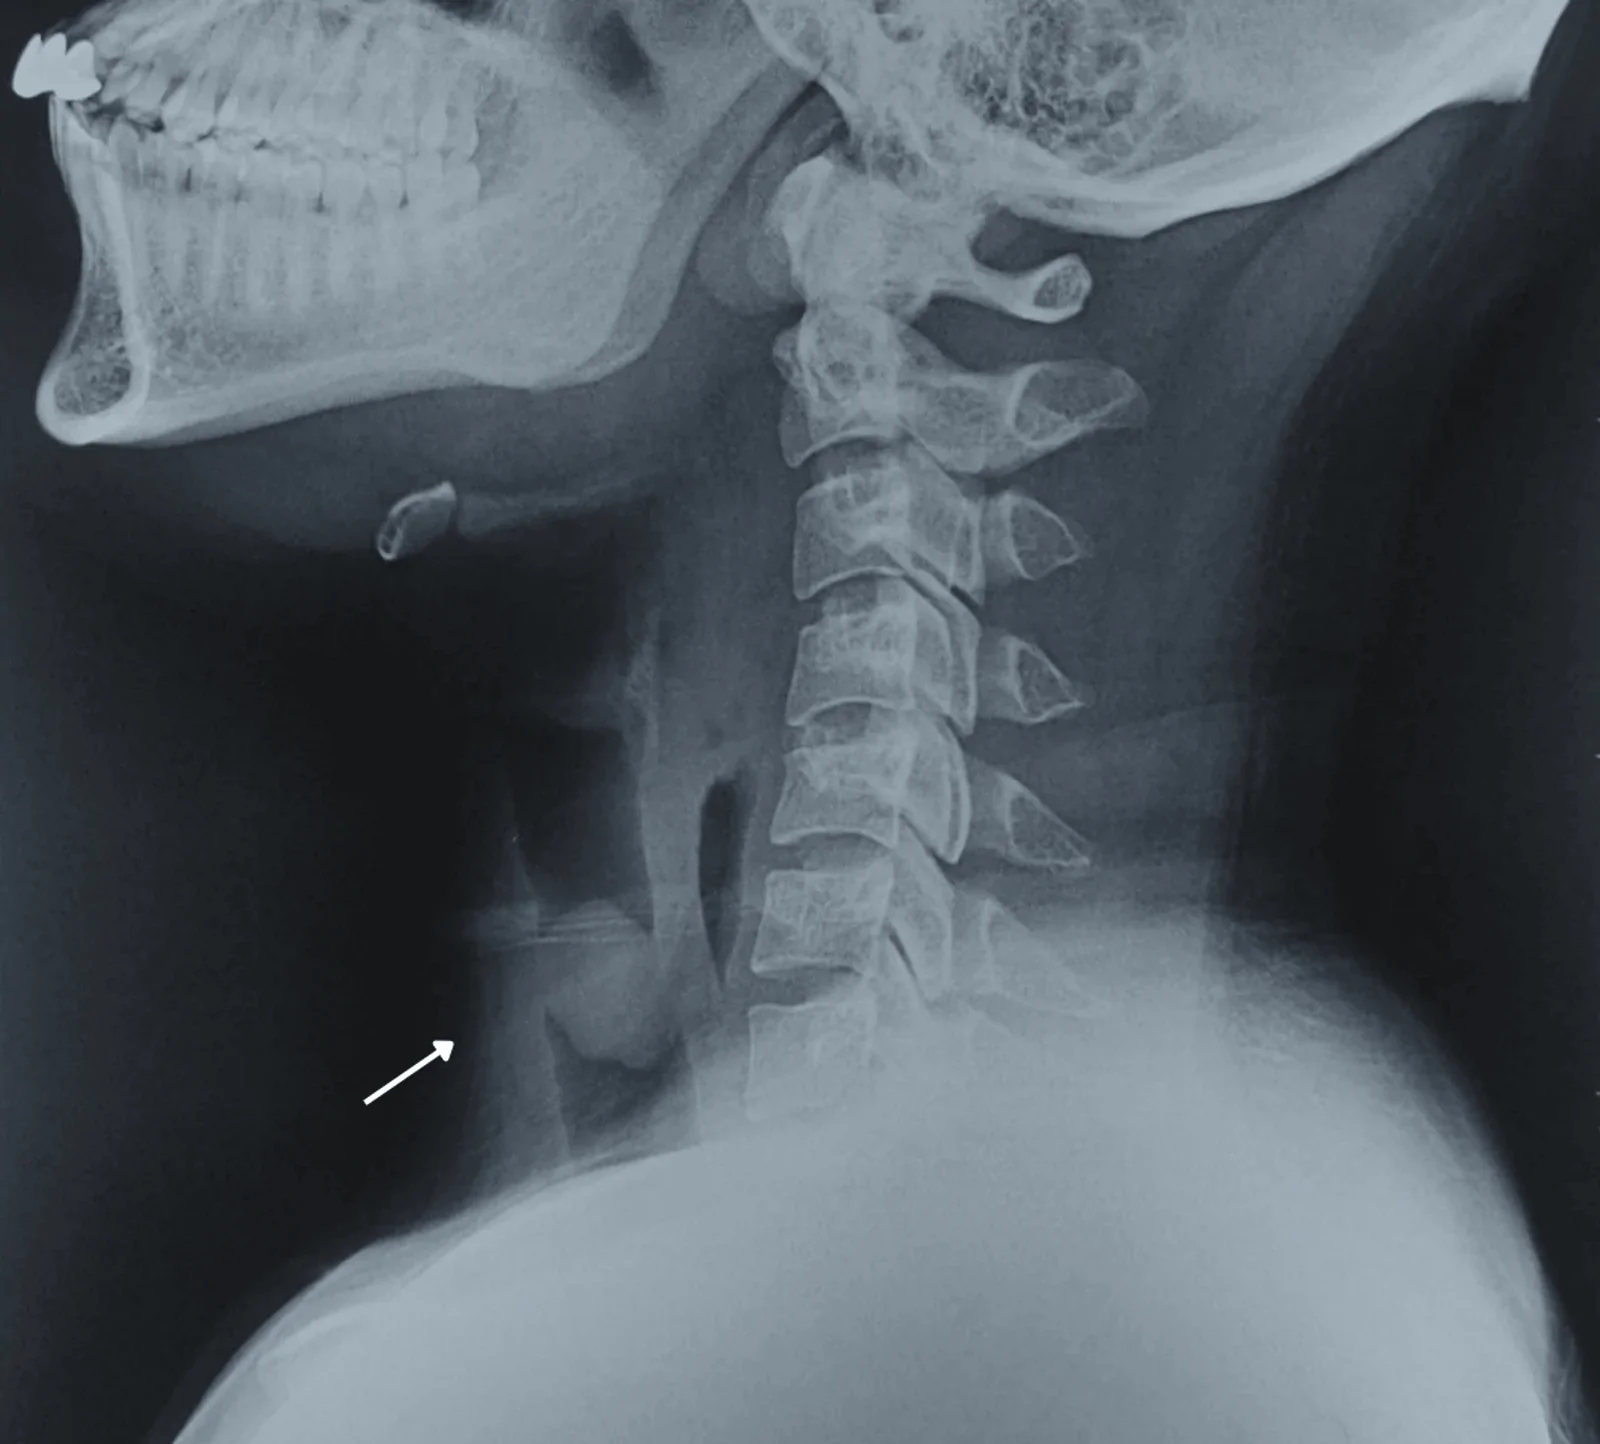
X-ray of the neck (lateral view) showing a soft-tissue opacity within the tracheal air column causing luminal narrowing at the C6–C7 level.
